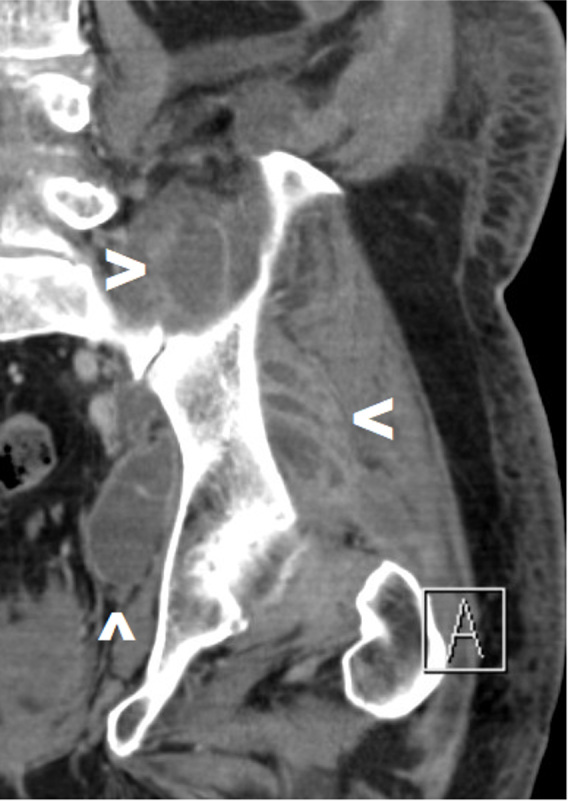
Pre-operative CT scan of a patient with SA of the left native hip due to hematogenous seeding with abscesses in the gluteal, iliopsoas, and external rotator regions (see arrows). A combination of a posterolateral approach and a Stoppa approach was deemed necessary.
